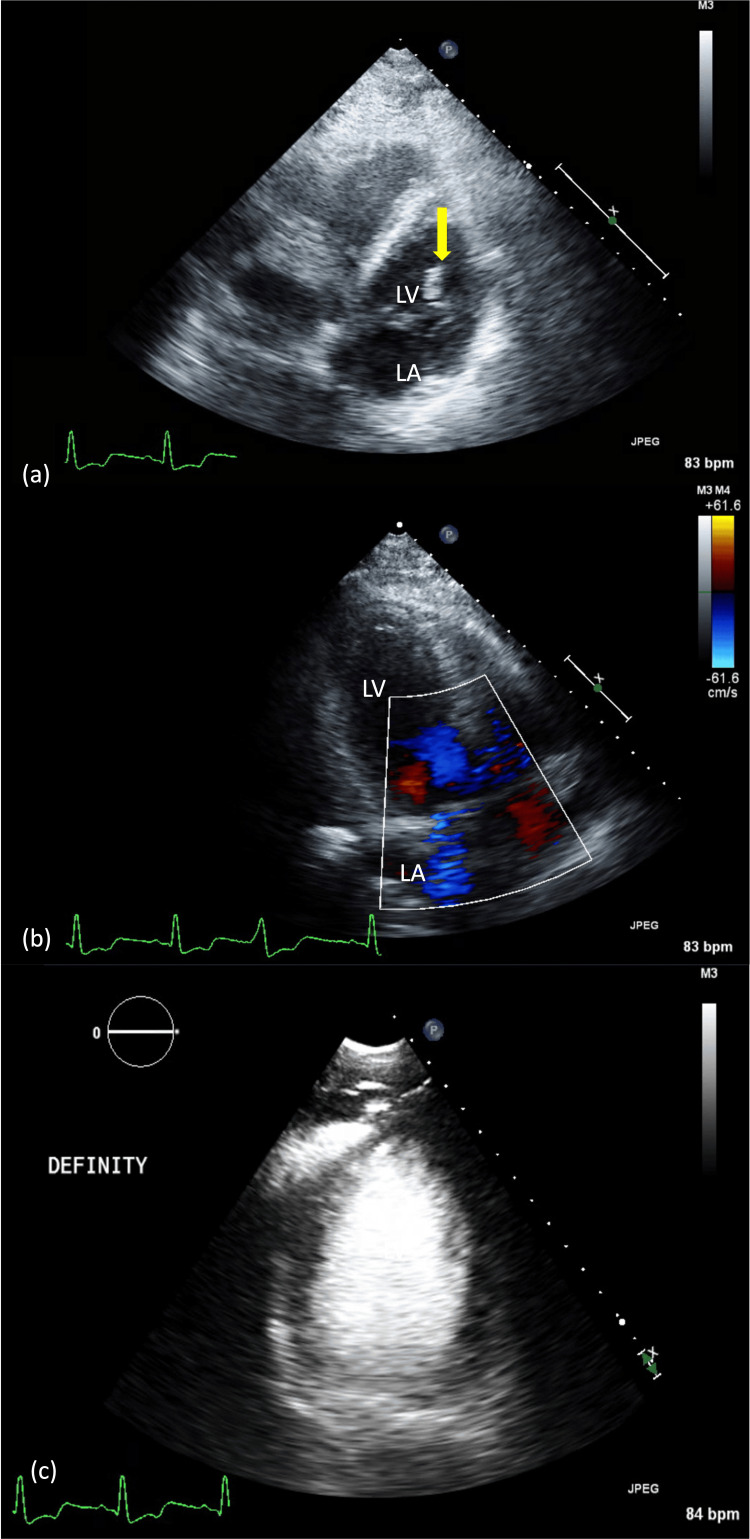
TTE views (a) Modified apical four-chamber view shows a linear echodensity (yellow arrow) within the LV cavity, appearing to be attached to the myocardium. (b) Modified apical three-chamber view demonstrates no evidence of associated MR or VSD. (c) Focused apical four-chamber view with echo contrast confirms the absence of an apical thrombus. LV, left ventricular; MR, mitral regurgitation; TTE, transthoracic echocardiogram; VSD, ventricular septal defect
